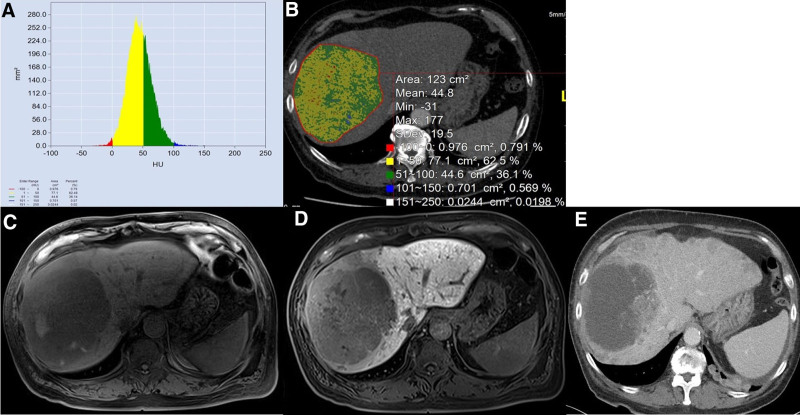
69-yr-old male patients with hepatocellular carcinoma not responding to atezolizumab plus bevacizumab. Computed tomography histogram analysis before atezolizumab plus bevacizumab (A and B) depicts relatively homogeneous vascularized HCC with mean-Hounsfield unit of 44.8, SD 19.5 Hounsfield unit measured in a region of interest covering the largest lesion. On EOB-DTPA pre-enhanced T1 weighted image (C) and hepatobiliary phase (D) the relative enhancement ratio ≥ 0.9 was defined. After 3 months of atezolizumab plus bevacizumab therapy, the new intrahepatic lesions are appeared, consistent with progression disease according to mRECIST (E). HCC = hepatocellular carcinoma.
